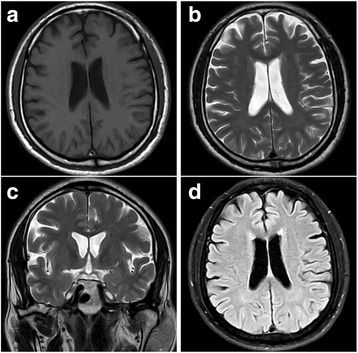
Brain magnetic resonance images (MRI) showed normal anatomy of brain without any mass or atrophic lesions. a T1-weighted axial image. b T2-weighted axial image. c T2-weighted coronal image. d T2-FLAIR axial image
